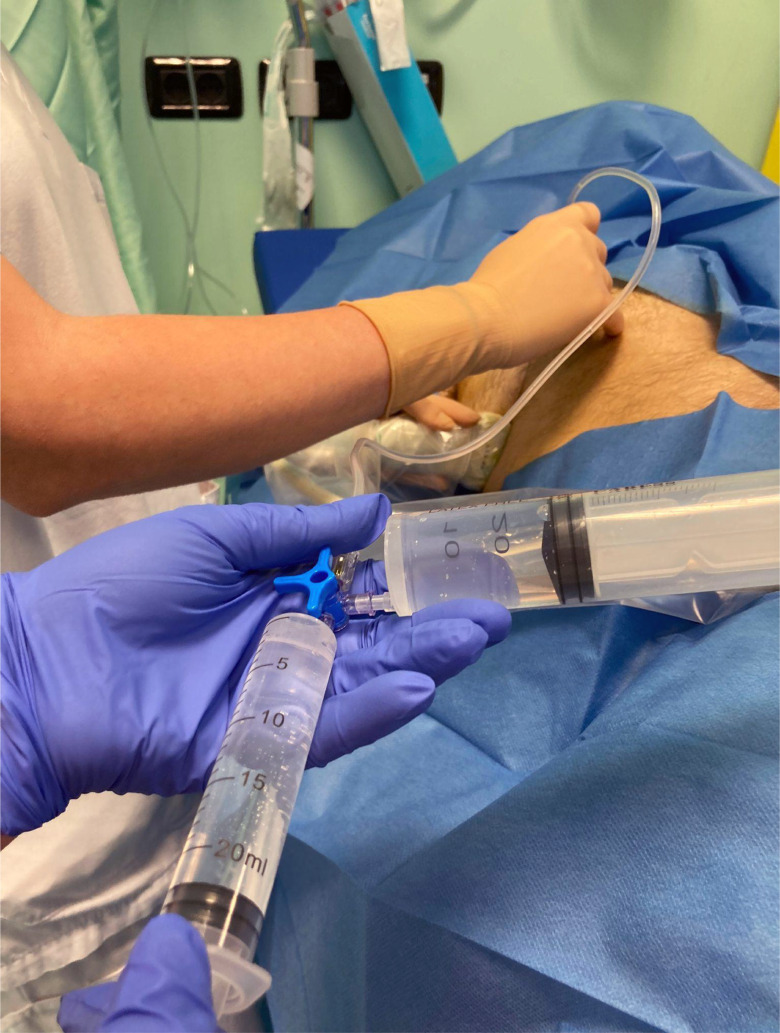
The technique is performed by two operators: the first operator controls the ultrasound probe and the position of the needle within the fascial planes via ultrasound guidance, and the second operator alternately injects diluted saline or ropivacaine according to the first operator’s instructions. The saline is injected to confirm the correct position of the needle in the fascial space; once the proper location of the needle is confirmed, the injection of ropivacaine is performed
